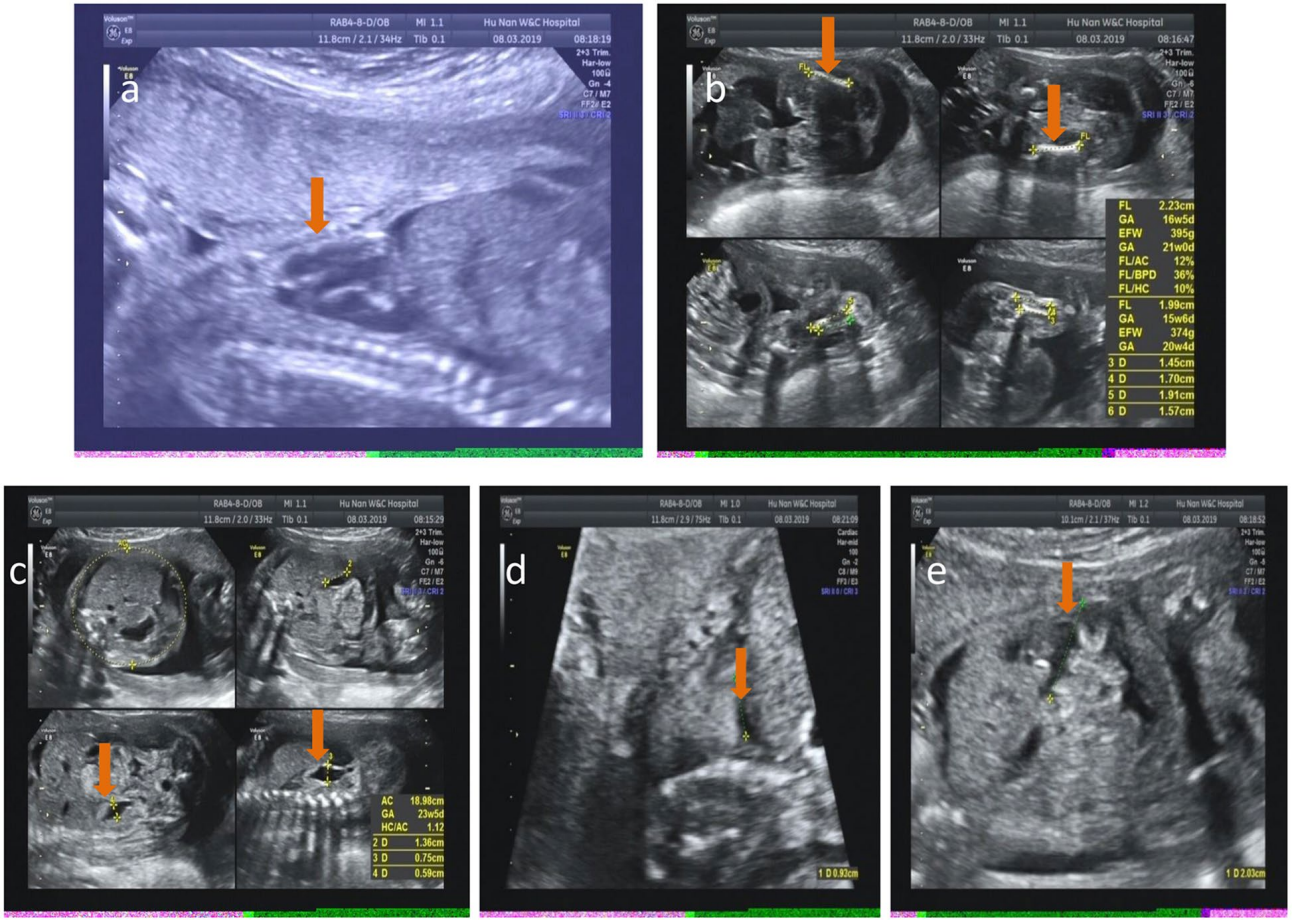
Ultrasound findings of the fetus in patient 1. Narrow thorax (a), shortened femur (b), bilateral pyelectasis (c), pleural effusion (d), and peritoneal effusion (e) are indicated by red arrows
